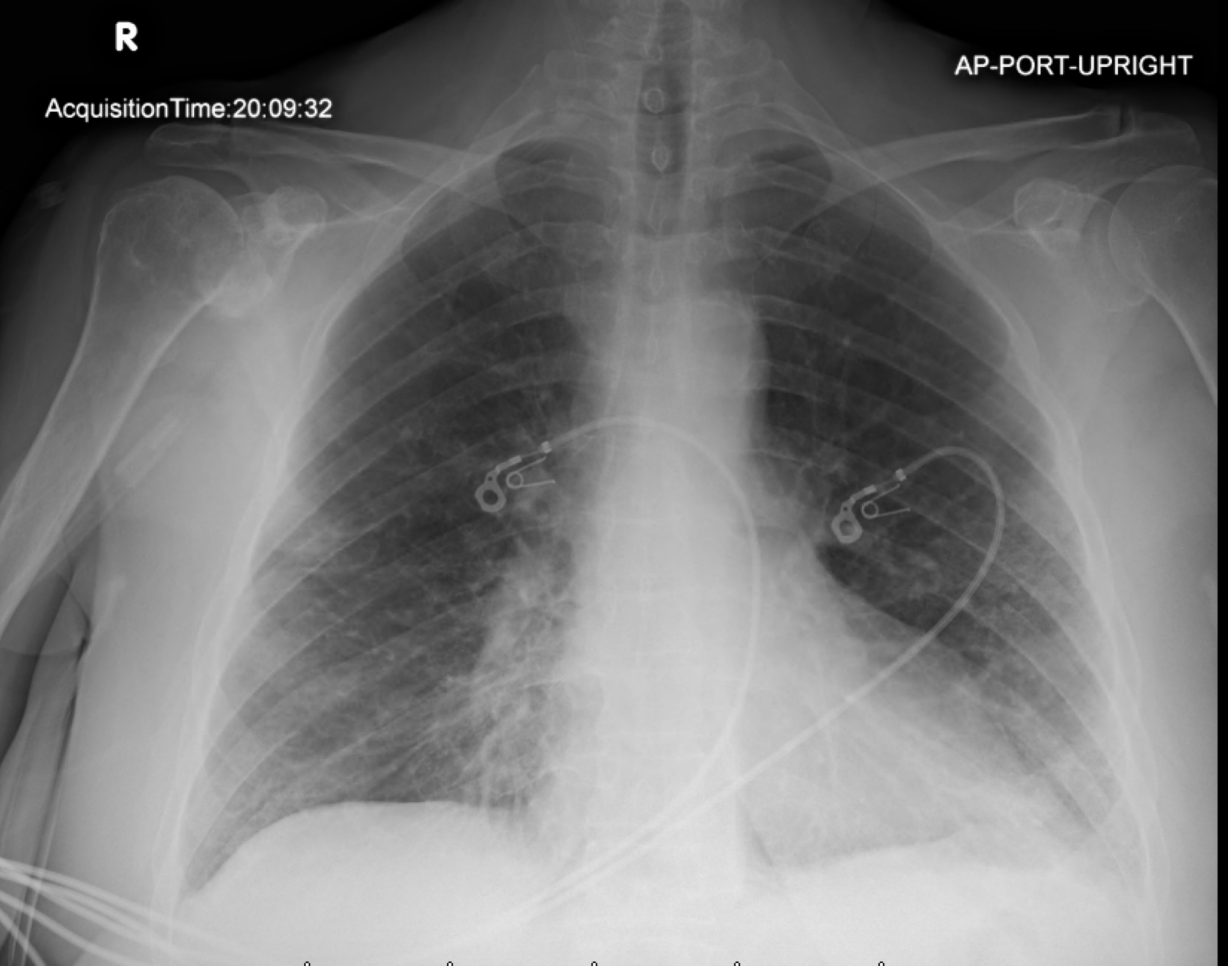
Initial Chest X-ray showing right mid-lung and bi-basilar airspace disease.
